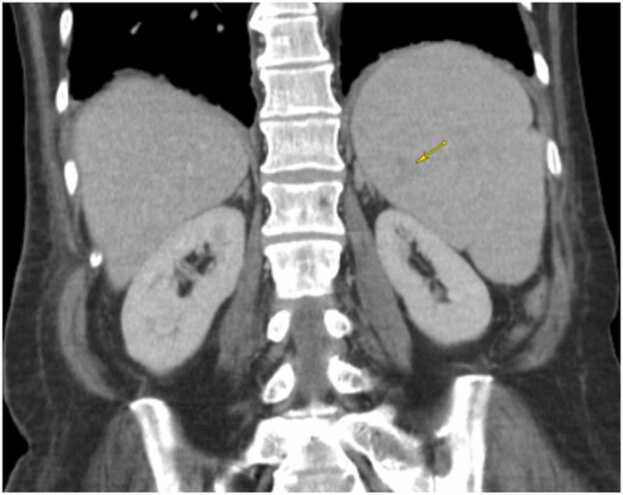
Computer Tomography (CT) Abdomen and Pelvis with intravenous contrast, Coronal view. Faint splenic wedge-shaped posterior ischemia or infarct (yellow arrow) and splenomegaly (left side) measuring 15.7 × 9.2 cm.
